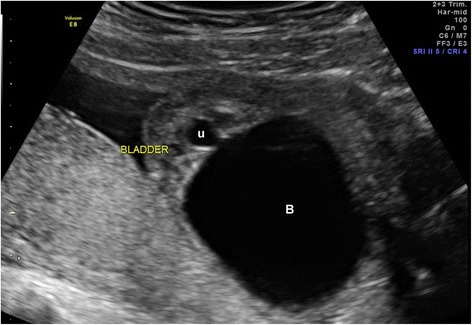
Ultrasound image of fetal bladder obstruction with the characteristic “keyhole” sign (*B: Bladder, u: urethra*)
